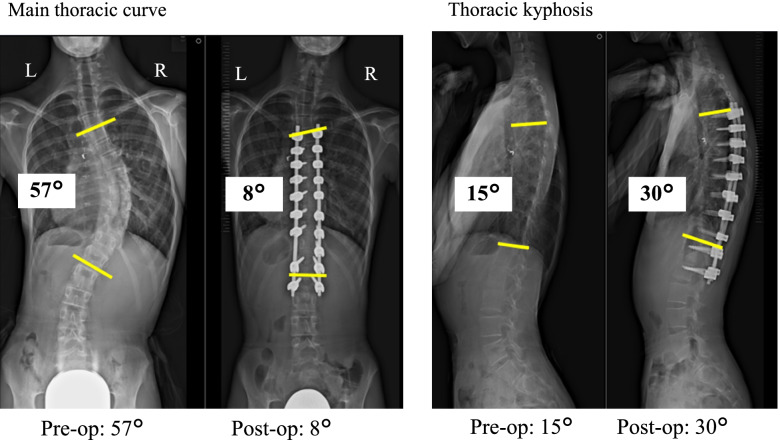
Pre- and postoperative radiographs. Radiographs showed that the correction rate of the main thoracic curve was 72% and thoracic kyphosis angle had increased by 15 degrees
